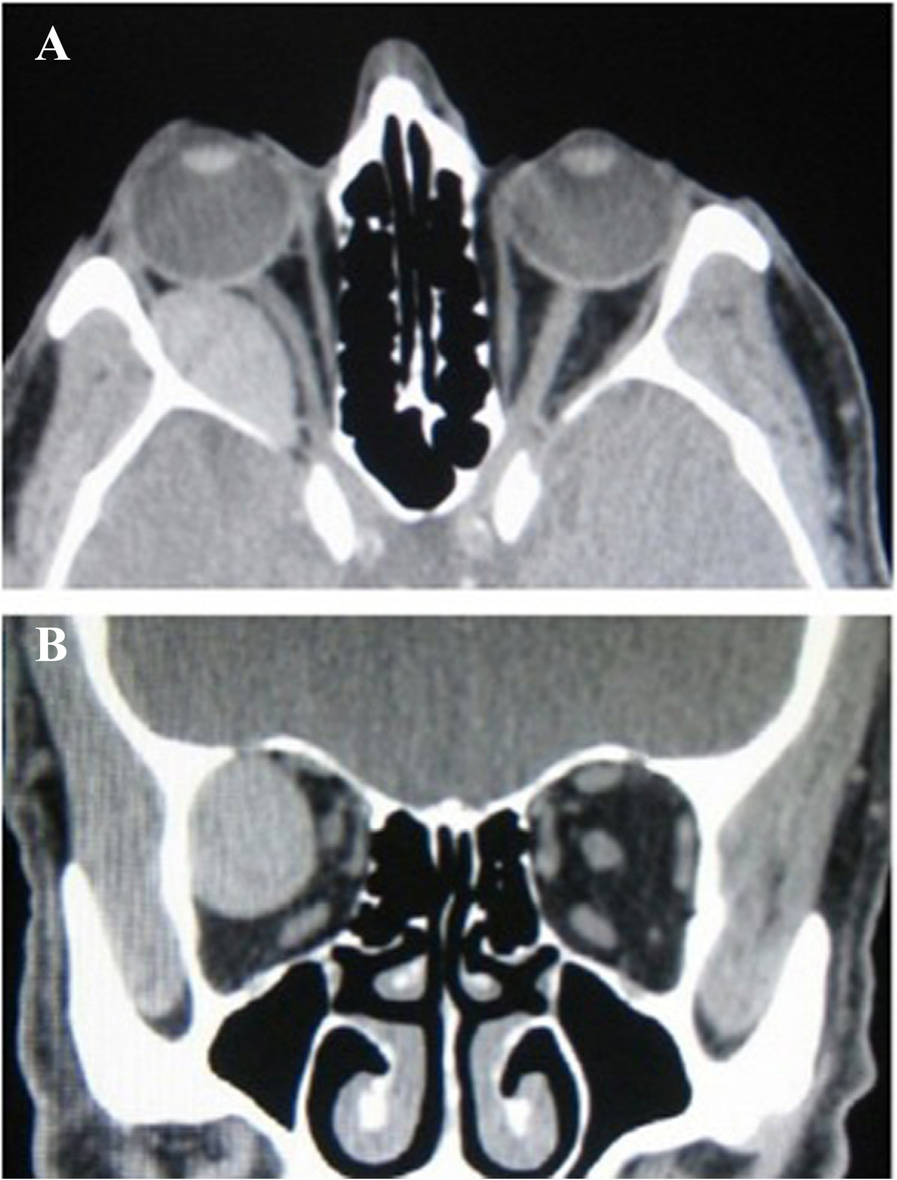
Axial (A) and Coronal (B) CT images of orbit revealed high density mass-like lesion in retrobulbar-extraconal part of right orbit consistent with subperiostal haematoma which displaces bulbus oculi, lateral rectus muscle and optic nerve.
